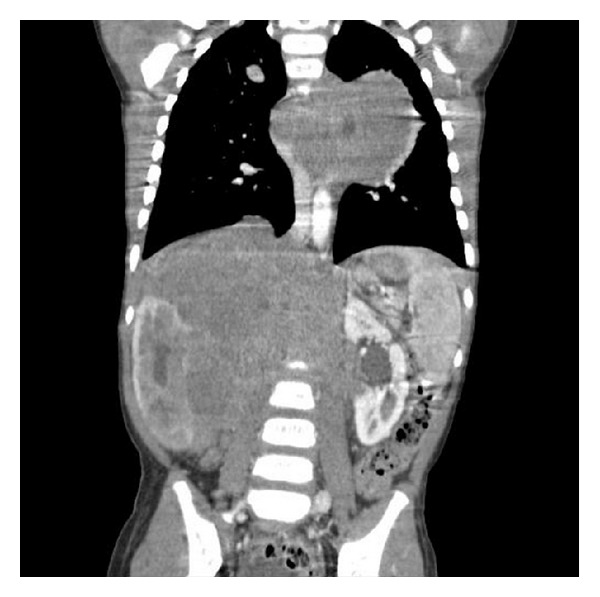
CT scan of abdomen and chest; large abdominal mass in right side and paravertebral mediastinal mass in left side.
